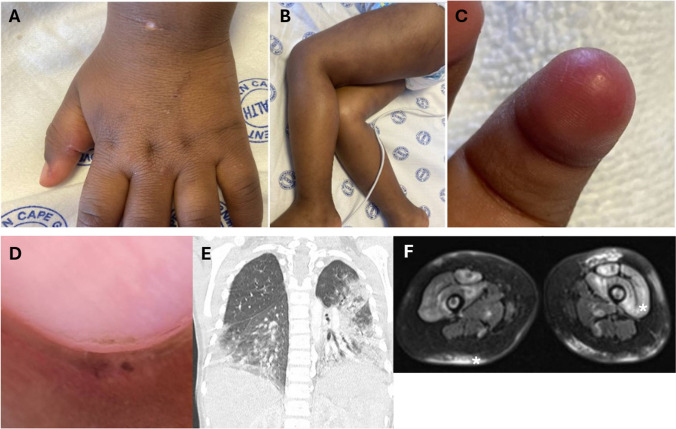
Clinical and imaging findings in patient 3. A Ulcer over the dorsal aspect of the wrist. B Hyperpigmented macular rash with mild excoriation. C Vasculitic rash over fingertips. D Subtle capillary loop dilation and small infarct visible on nail bed capillaroscopy. E Coronal CT chest demonstrates bilateral lower lobe and lingula confluent ground glass opacification with left lower lobe traction bronchiectasis. F MRI showing T2 axial and coronal fat sat images that demonstrate extensive high T2 hyperintense signal abnormality in the muscles and subcutaneous tissues of the thighs bilaterally (white asterisks)
